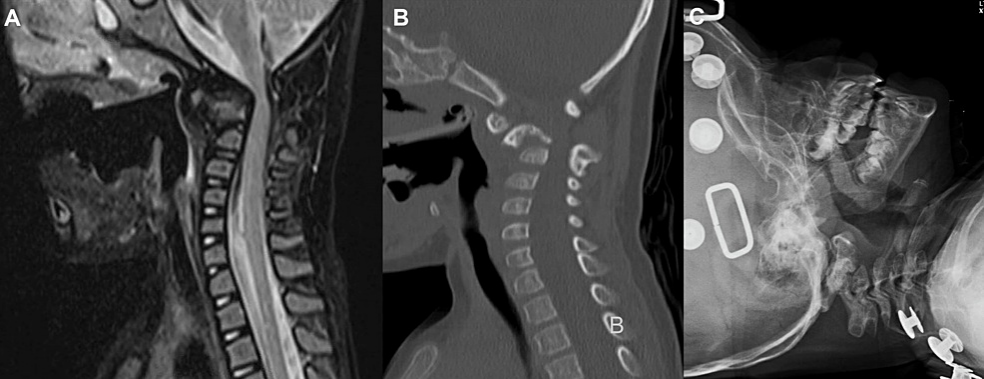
Preoperative. (A) T2 MRI brain showing atlantoaxial subluxation worsening. (B) Neck CT demonstrating stable destructive changes involving atlantoaxial level and causing narrowing and kinking of the spinal cord. (C) Neck X-ray showing a deformed C2 vertebra with a further widening of the atlanto-odontoid interval and narrowing of the foramen magnum. MRI, magnetic resonance imaging; CT, computed tomography
